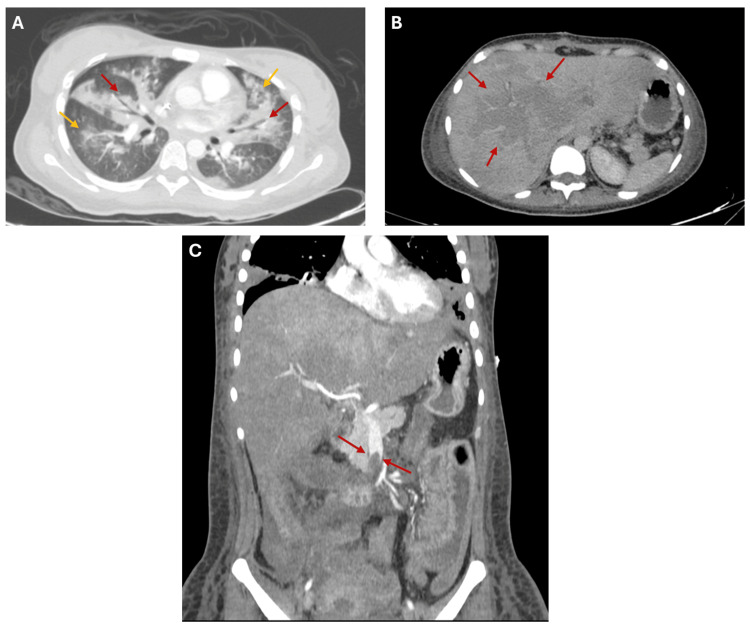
Chest and abdominal computed tomographies. A. Contrast-enhanced chest computed tomography: Bronchovascular thickening (red arrows) and peribronchial consolidation (yellow arrows), suggestive of pulmonary edema.
